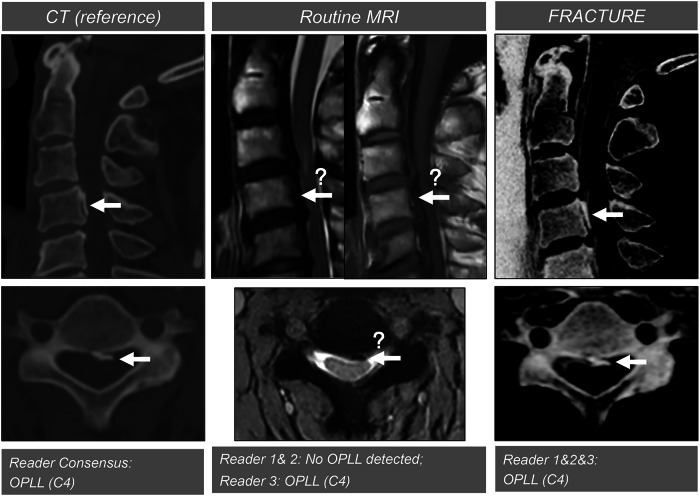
Examples of three imaging protocols for bony cervical spondylosis. From left to right, CT, conventional MRI, and FRACTURE sequences were shown. Conventional MRI sequences underestimated the bony abnormalities, while FRACTURE could be optimized to closely match the CT-diagnosed findings
